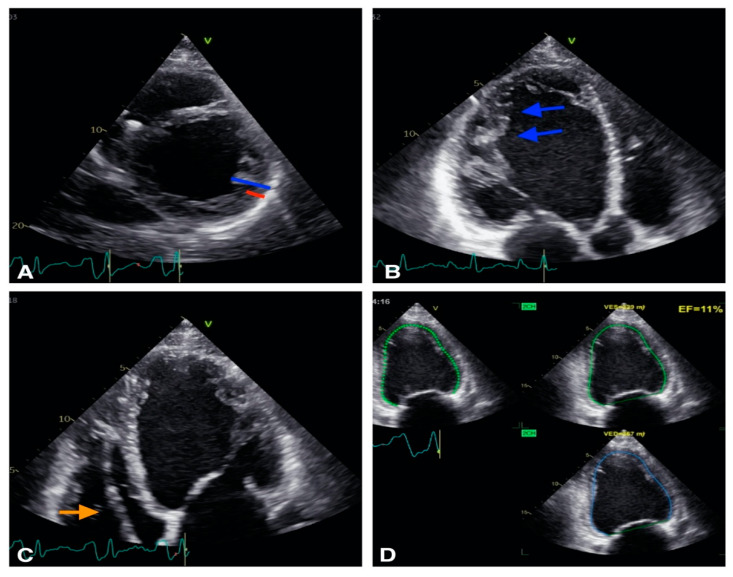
Example of a patient with non-compaction cardiomiopathy. (A): 2D short axis view, the non compaction myocardium (blue) is twice thicker than the compacted myocardium (red). (B): 2D apical 3 chambers view, trabeculations are visible (blue arrows). (C): 2D apical 4 chambers view: defibrillator lead is visibile in right ventricle (orange arrow). (D): Biplane EF is calculated at 11% showing severe LV systolic dysfunction.
